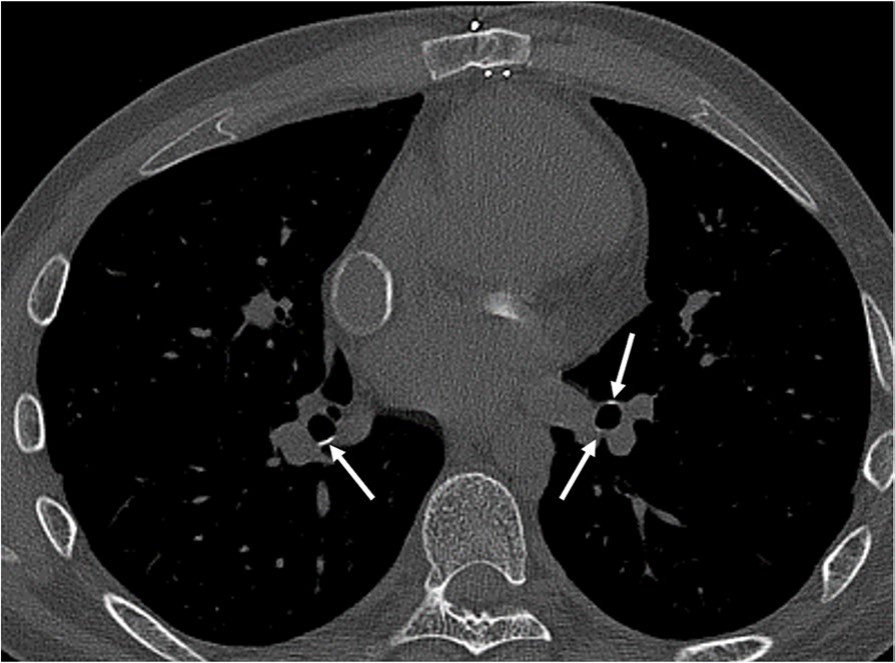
Tracheobronchial calcification associated with long-term warfarin therapy after pulmonary artery banding and ligation of patent ductus arteriosus in a 27-year-old man. Bone window CT image shows that calcifications can be identified along the bronchi (arrows)
